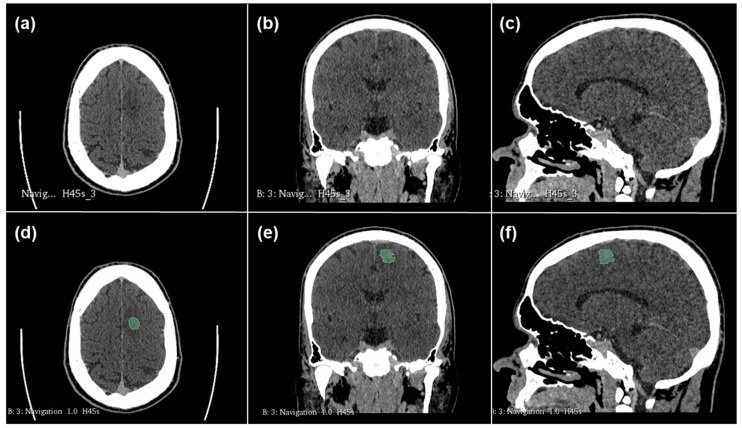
Case of low-grade glioma (LGG). Patient aged 45 years old with oligodendroglioma localized in left frontal lobe. Images (a–c): original CT images before segmentation. Images (d–f): semi-automatic segmentation with 3D Slicer (areas marked in green).
